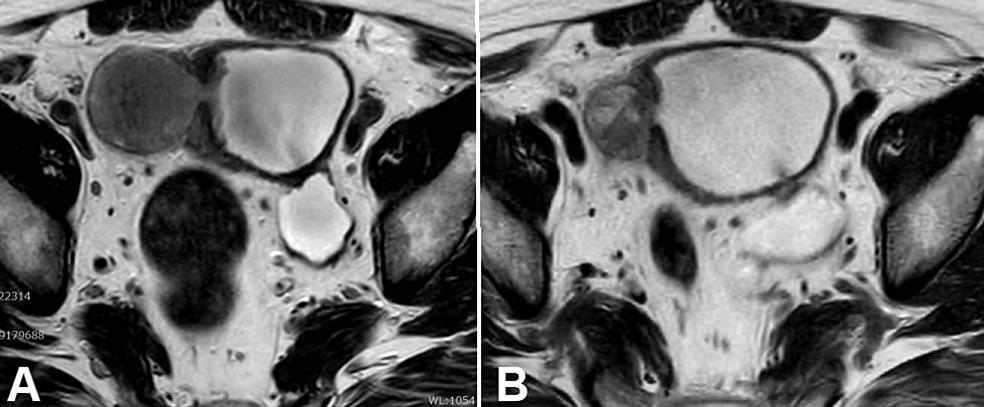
The status of bladder tumors before and after neoadjuvant chemotherapy. Magnetic resonance imaging showed a bladder tumor within a bladder diverticulum. (A) Tumor before platinum-based neoadjuvant chemotherapy. (B) After two cycles of platinum-based neoadjuvant chemotherapy, the tumor was found to have decreased in size by more than 50%.
